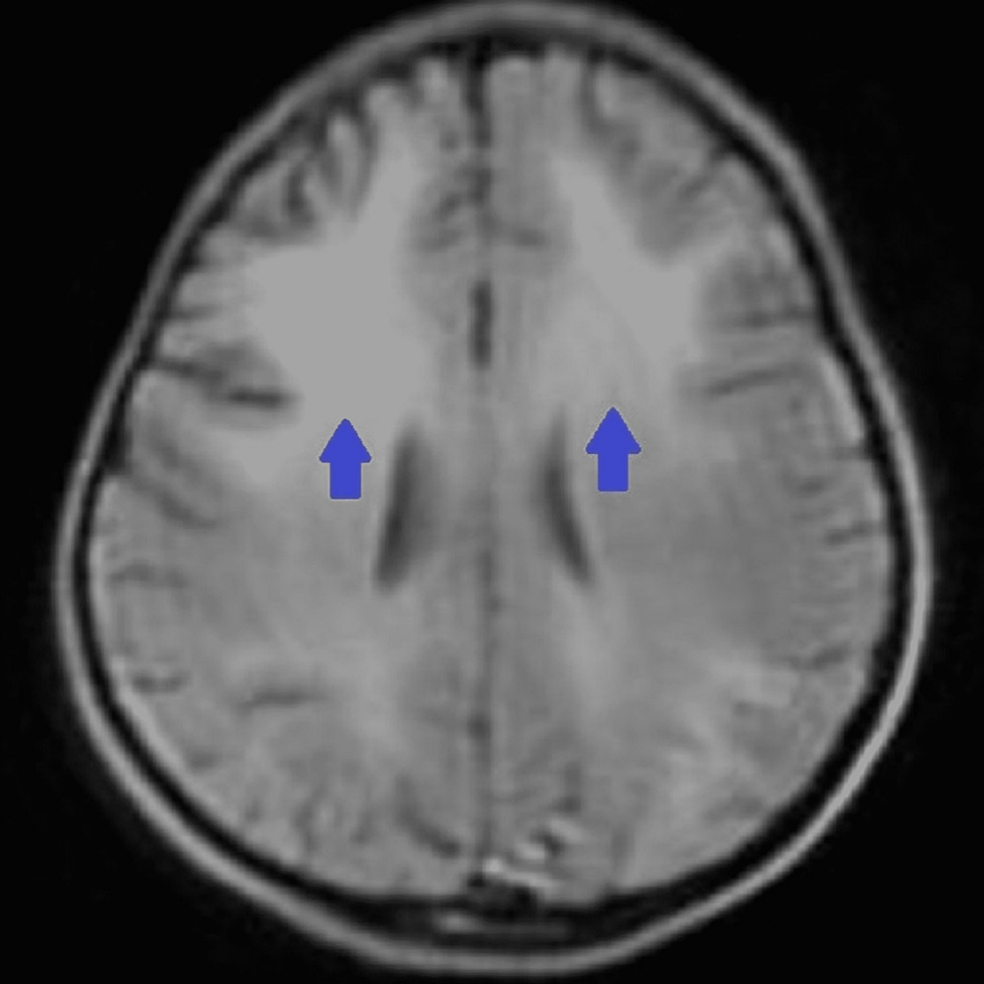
FLAIR image shows diffuse symmetrical, bilateral hyperintensities with preponderance of frontal lobes (blue arrows). FLAIR: fluid-attenuated inversion recovery
